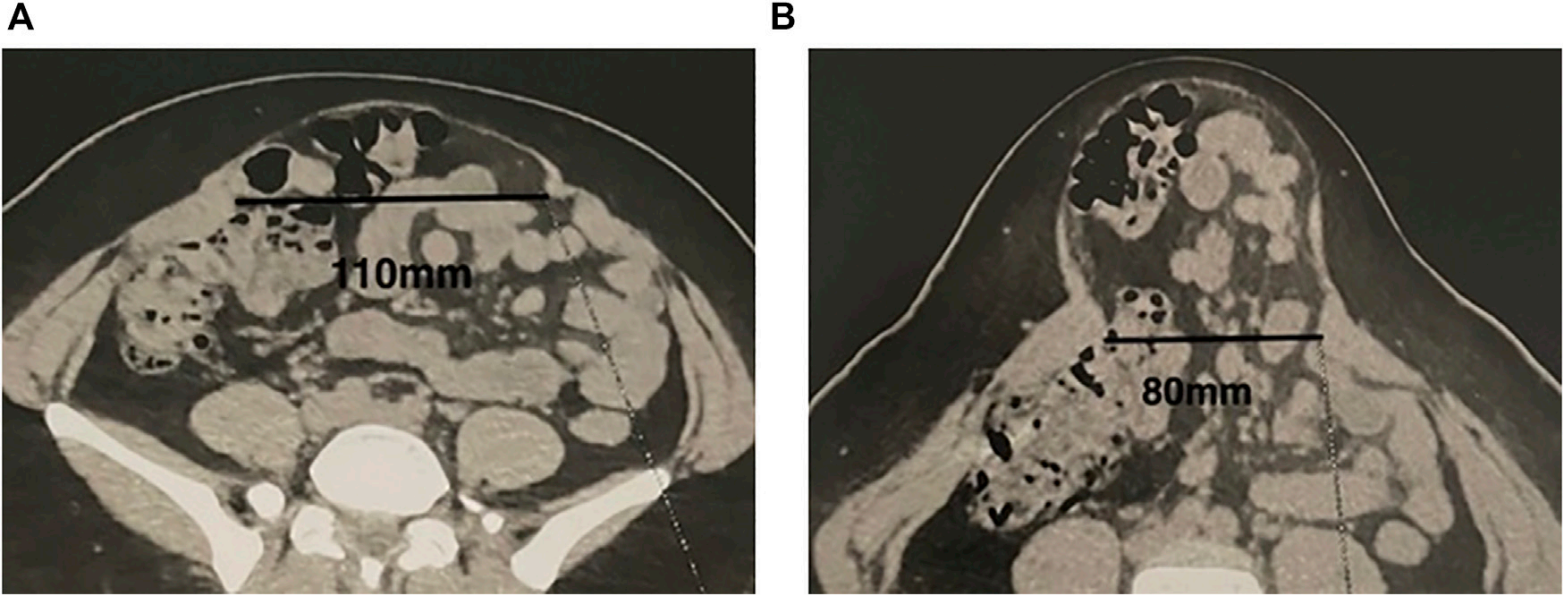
Measuring the width of divarication on the CT scan at rest (A) and at straight leg raise (B).
